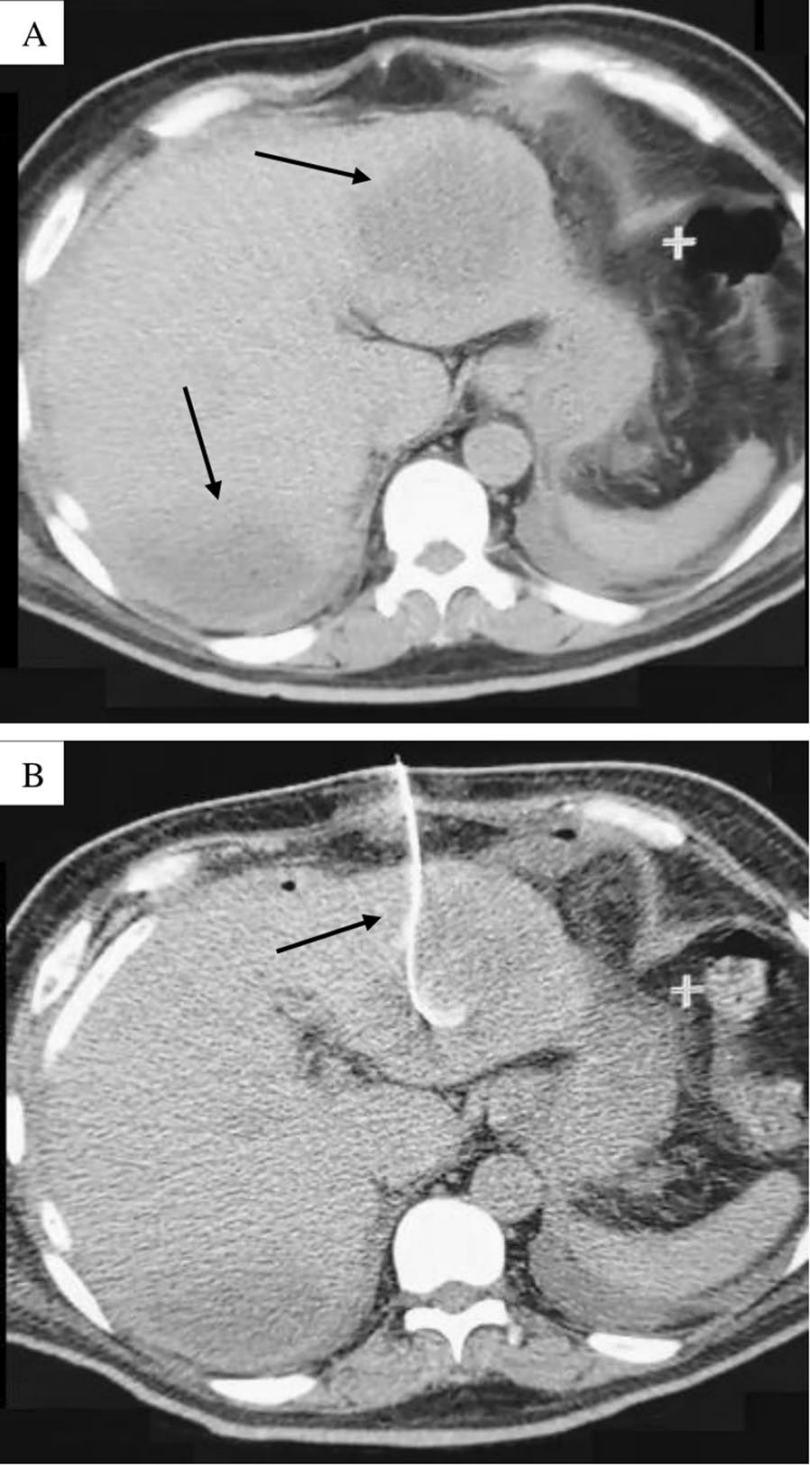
Computed tomography. A Axial section of abdominal computed tomography scan without contrast administration showing an enlarged liver (caudal skull diameter: 18 cm), at least two images were located in segments II and VIII of rounded morphology, regular edges, hypodense (Hounsfield Units: 20), the largest with 150 cc volume, likely related to liver abscess; B axial section of abdominal computed tomography scan without oral or intravenous contrast administration, showing the placement of a catheter for drainage of the liver abscess located in segment II
